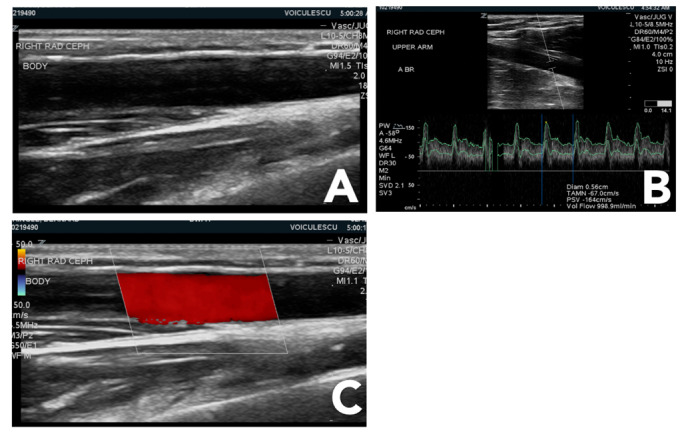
Ultrasound modes used for vascular access examination. A) B-mode (brightness); B) D-mode (Doppler); C) C-mode (color); Figures courtesy of Dr. Adina Voiculescu.
